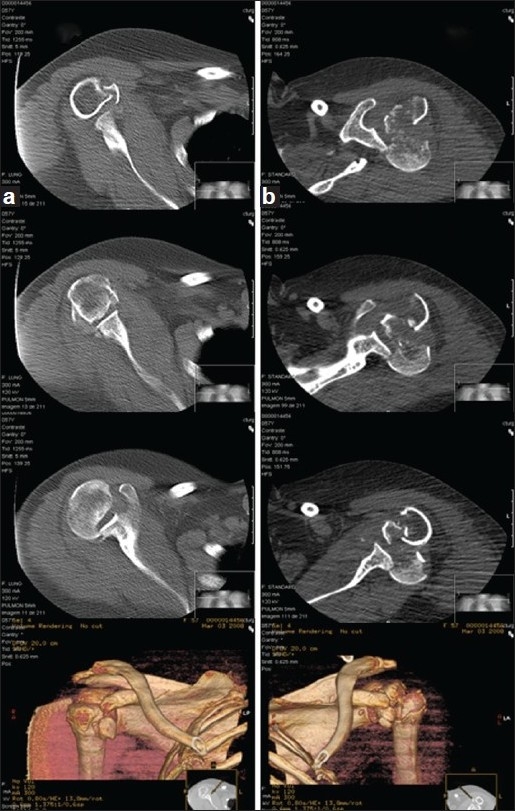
CT scan of both shoulders [right- (a); left- (b)] confirming a bilateral posterior four-part fracture-dislocation of the shoulder
